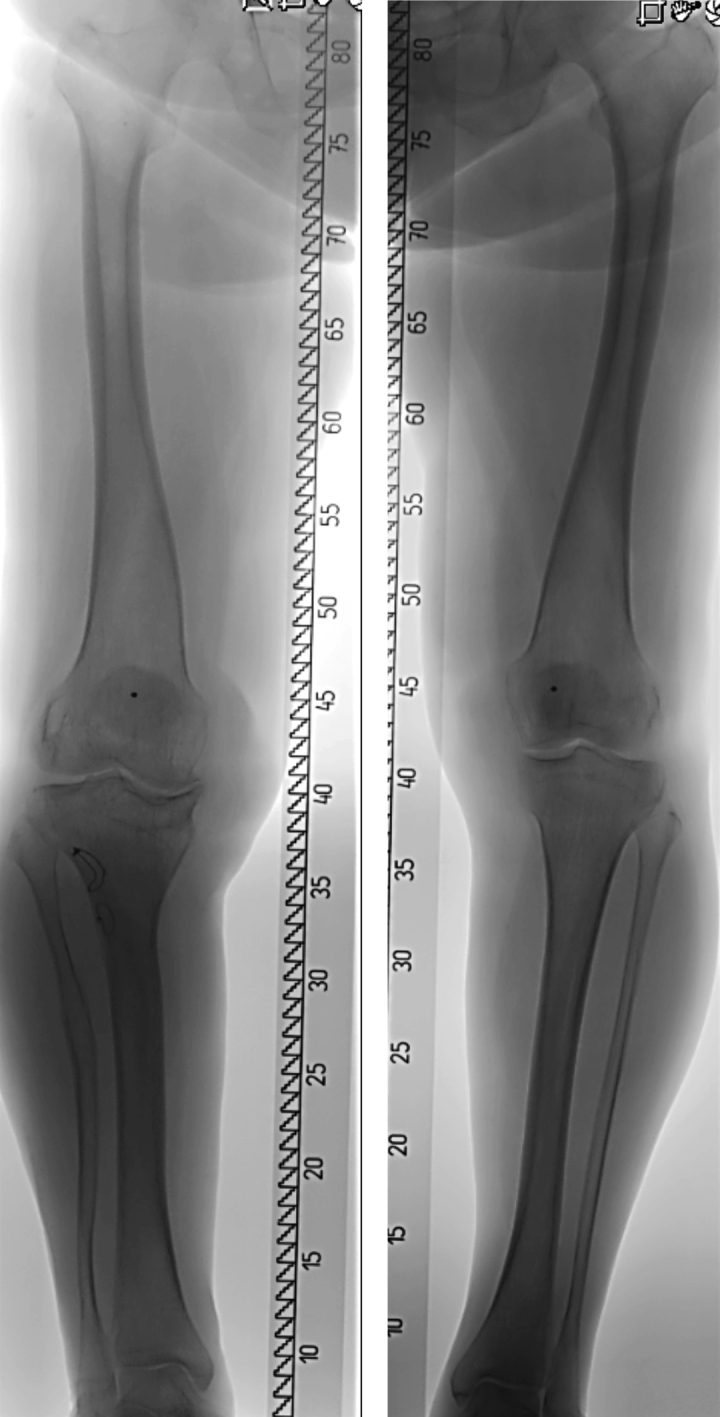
Long-leg standing radiographs at 65 years follow-up, showing slight varus of the right knee and leg length discrepancy of 1.5 cm with the right leg shorter.
